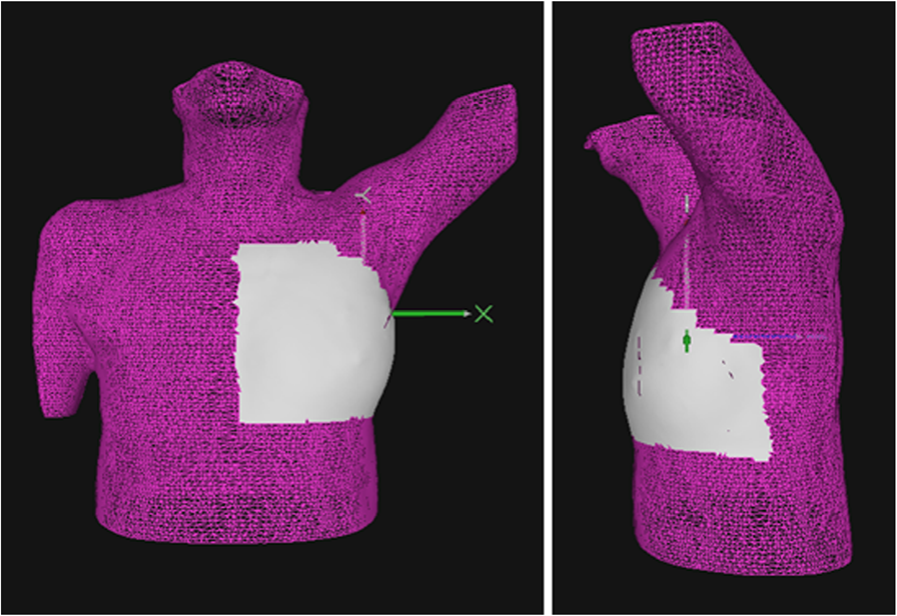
The 3D body surface of the patient from the planning CT is represented by a pink mesh. The region of interest (ROI) used for monitoring the patient surface is shown in grey
